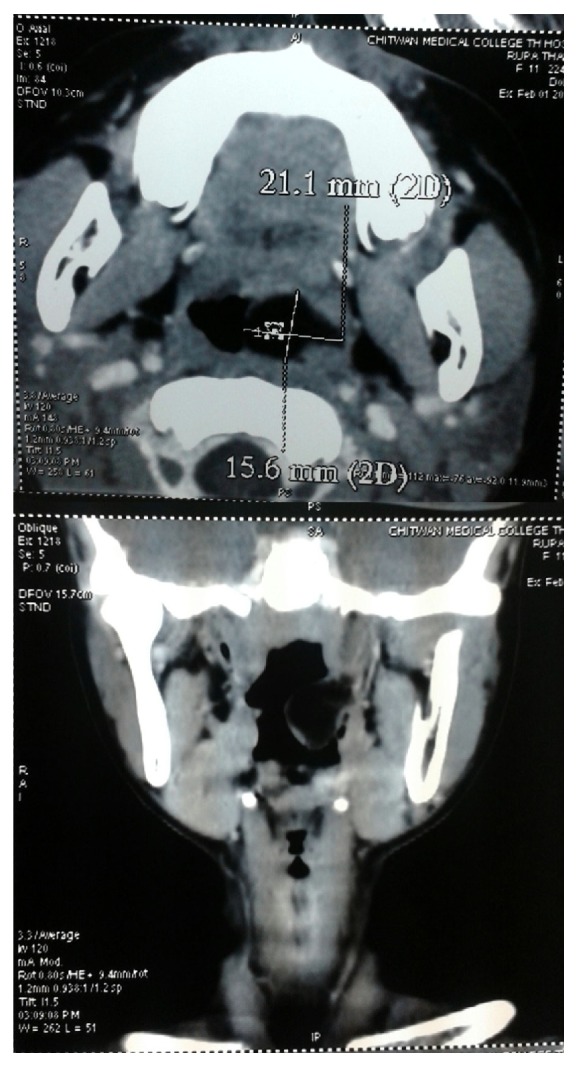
CT scan showing the soft tissue mass in the nasopharynx (nasopharyngeal mass) (axial and coronal view).
